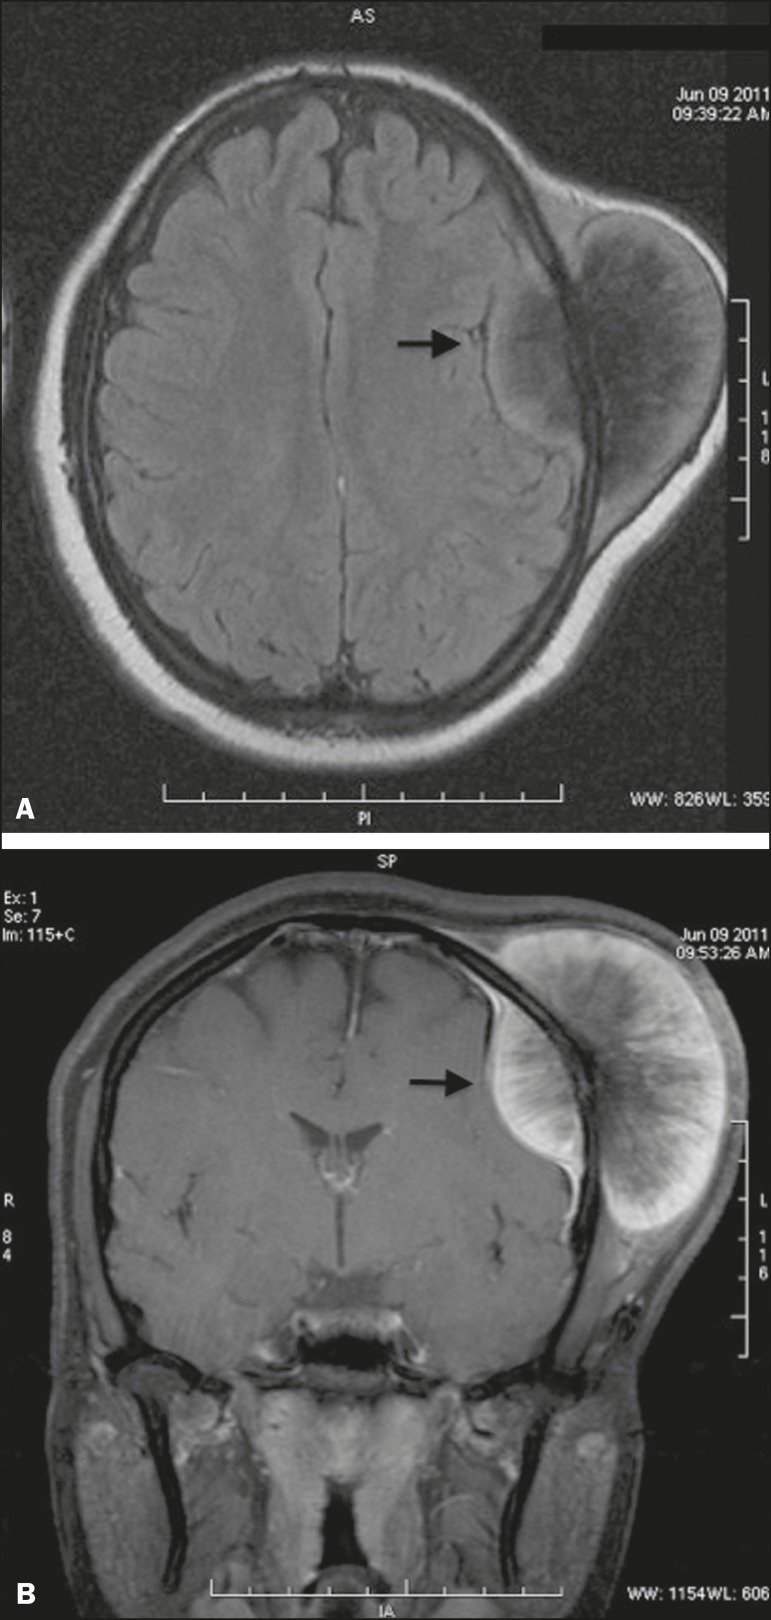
Contrast-enhanced magnetic resonance imaging of the skull-a axial
